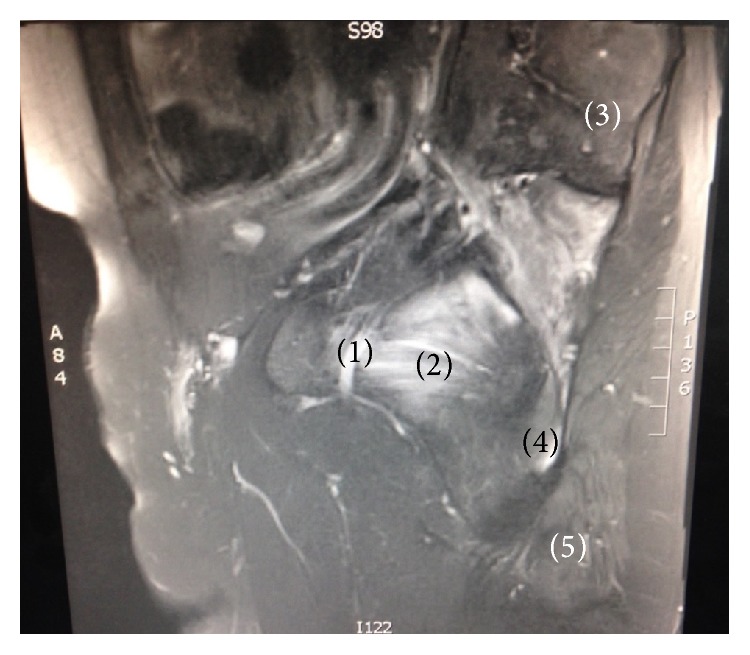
Oblique parasagittal section of T2-weighted MRI displaying passage of the right sciatic nerve above the piriformis muscle. (1) Sciatic nerve. (2) Piriformis. (3) Sacroiliac joint. (4) Ischium. (5) Ischial tuberosity.
